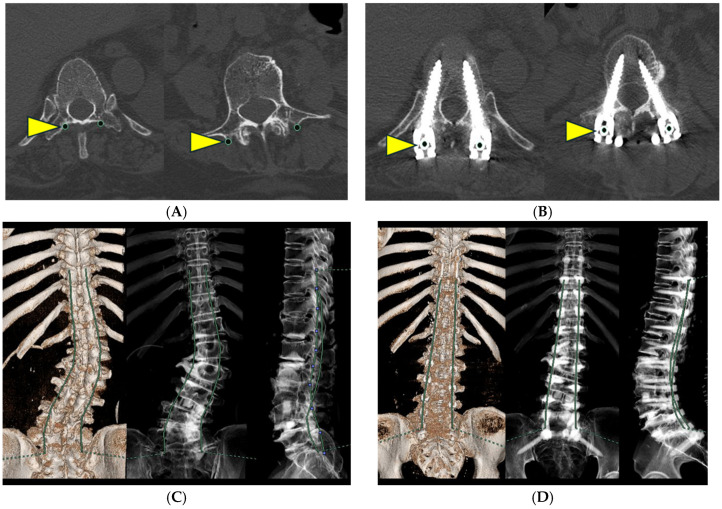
Axial CT images used for plotting rod trace length (RTL) and three-dimensional reconstruction of PSTL from plotted points. (A) Planned pedicle screw insertion points were bilaterally plotted on axial CT images obtained after lateral lumbar interbody fusion (LLIF) to calculate the Pre-correction RTL (Pre-RTL). (B) Actual pedicle screw head centers were bilaterally plotted on axial CT images obtained after posterior correction to calculate the Post-correction RTL (Post-RTL). (C) The RTL before posterior correction (Pre-RTL) was calculated by connecting the planned pedicle screw insertion points from T9 to S1 after lateral lumbar interbody fusion (LLIF). (D) The RTL after posterior correction (Post-RTL) was calculated by connecting the actual screw head centers from T9 to S1 after posterior correction. Yellow arrowheads and black circles: plotting points; Green line: Rod trace length (RTL).
